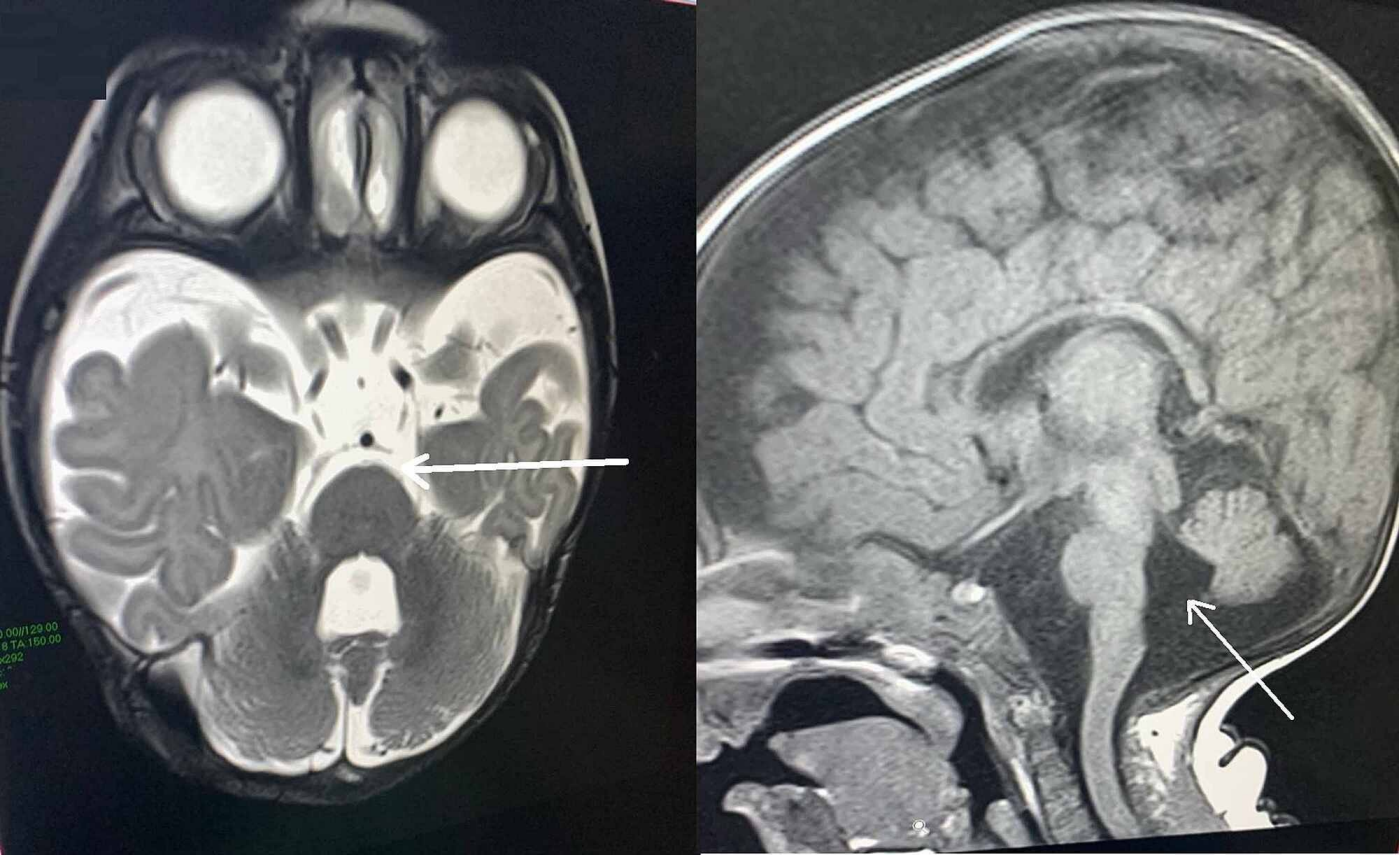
Magnetic resonance imaging of the brain showing mild periventricular leukomalacia, benign external hydrocephalus with the suggestion of Blake's pouch cyst Arrows showing Blake's pouch cyst
